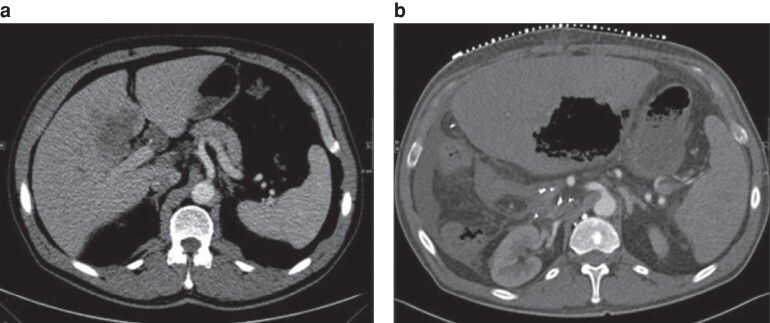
Class II position change of the coeliac trunk in-between the preoperative scan and the postoperative scan (32 days after right-sided hemihepatectomy) a Preoperative image. b Postoperative image. Axial plane. Note the gas-filled hepatic lesion in the remaining left lobe, compatible with hepatic gangrene, in the postoperative image. Postoperative imaging was performed for CT-guided drainage.
